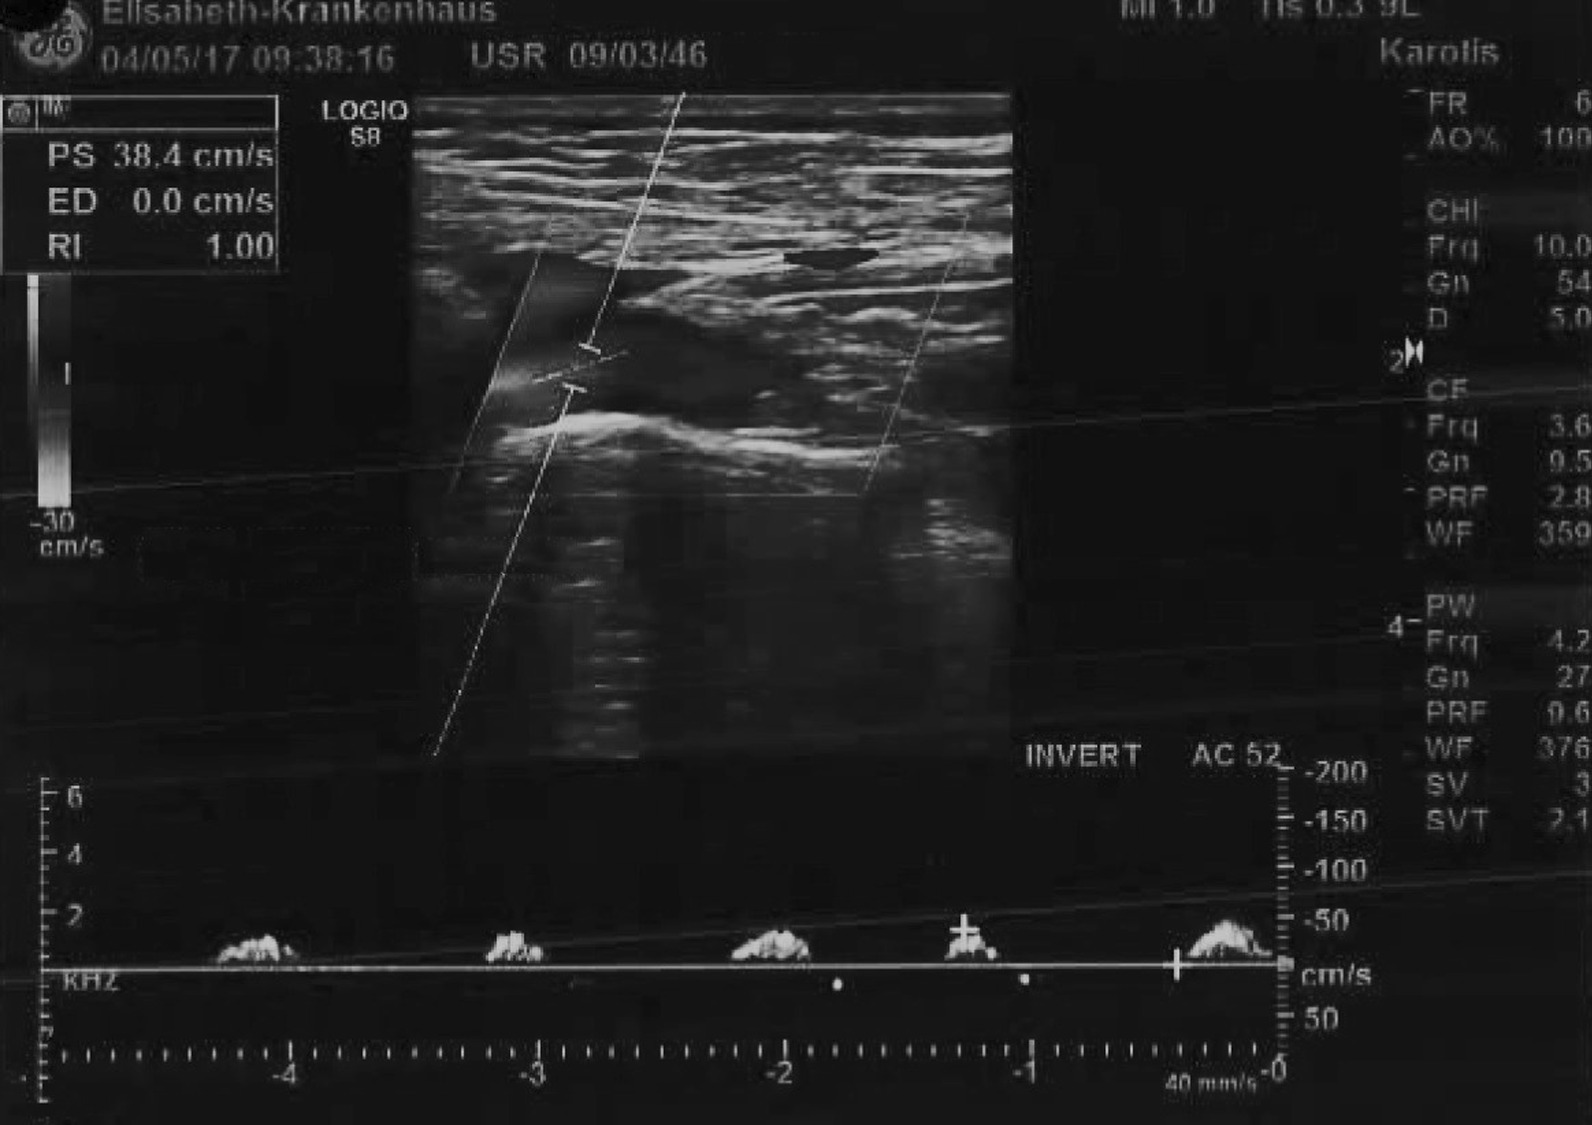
Sonography of the left proximal subclavian artery, showing a low flow profile (0.38 m/s) with the subsequent occlusion
